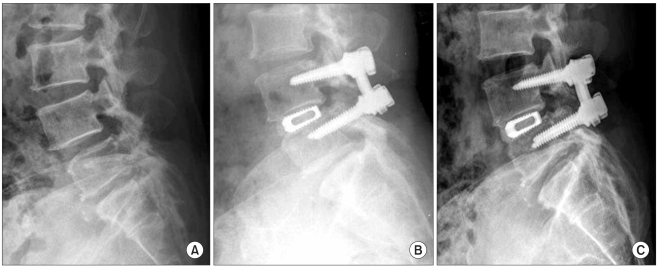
A 51-year old female with spinal stenosis at L4-5. (A) The preoperative lateral view shows a decreased disc height at L4-5. (B) The lateral view after surgery shows restoration of the disc height. (C) At 3 year after surgery, the lateral radiograph shows solid fusion and maintenance of reduction.
